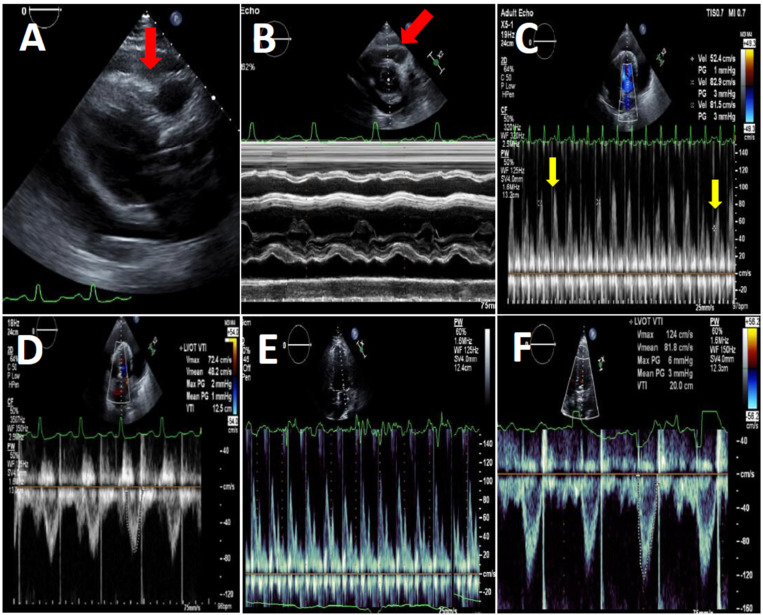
Transthoracic echocardiography of a COVID-19 patient before (panels (A–D)) and after pericardiocentesis (panels (E,F)). (A)—Parasternal long axis view, diastolic RV free wall collapse (red arrow) due to high pressure in the pericardial space, due to a large circumferential pericardial effusion. (B)—Short axis view M-Mode at the level of the aortic valve showing diastolic collapse of the RV free wall (red arrow). (C)—Apical 4-chamber view PW Doppler of the mitral flow showing respiratory variations between beats (yellow arrows). (D)—Apical 4-chamber view PW Doppler of the left ventricular outflow tract systolic flow showing low VTI, indicating low stroke volume. (E)—Apical 4-chamber view PW Doppler of the mitral flow showing normalization of mitral flow pattern. (F)—Apical 4-chamber view PW Doppler of the LVOT systolic flow showing normal VTI, indicating normal stroke volume.
